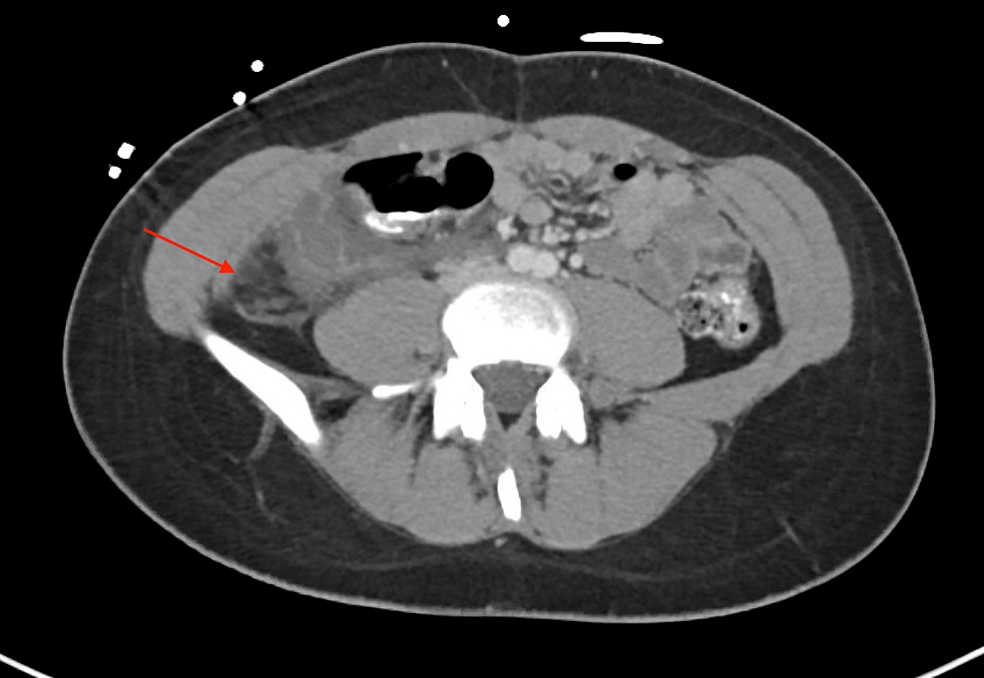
Abdominal CT scan with IV contrast revealing an inflammatory process involving the cecum and ascending colon.
